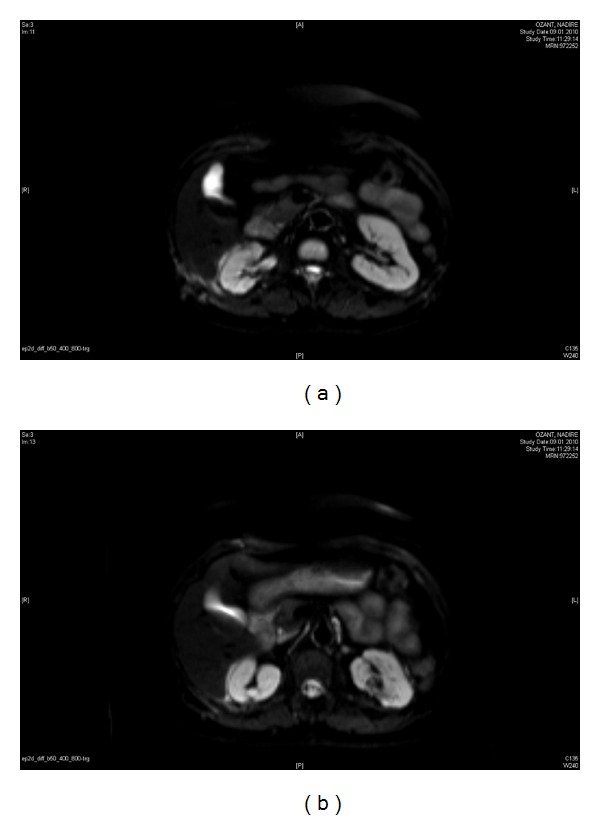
MRI scans performed 2 years after surgery, documenting no tumoral lesion.
